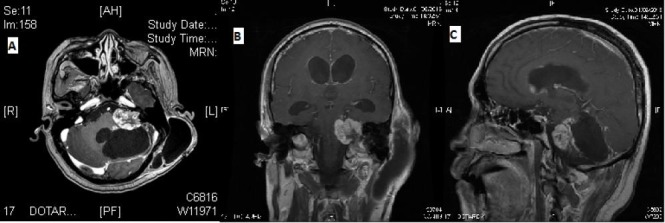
Magnetic resonance imaging performed post-surgery and re-irradiation A) Axial T1-weighted contrast-enhanced image; B) Coronal T1-weighted contrast-enhanced image; C) Sagittal T1-weighted contrast-enhanced image
